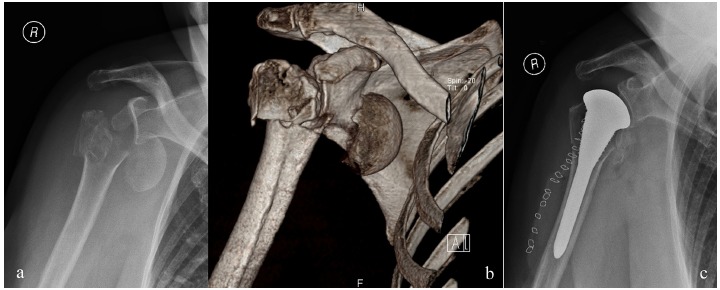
X-ray (a) and 3D reconstruction CT (b) of a 4-part humeral head fracture treated with hemiarthroplasty (c).
